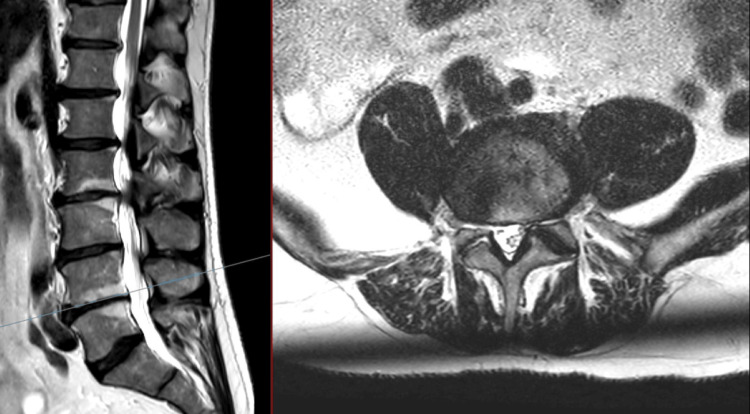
T2 MRI of the lumbar spine: sagittal (left) and axial (right) at L4/5 No compression of the cauda equina was noted. There was no evidence of compression of the right L5 nerve root.
